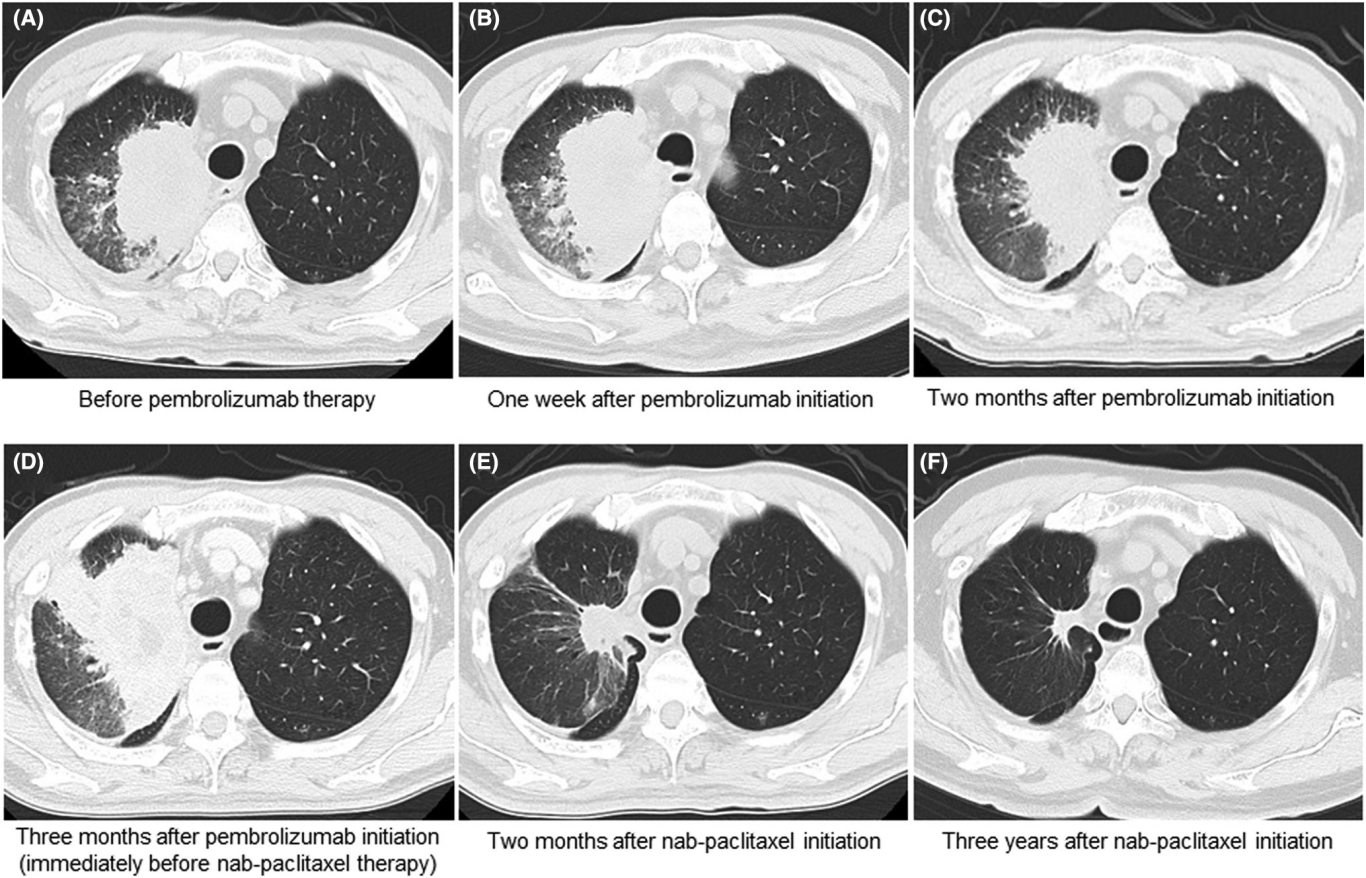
Representative CT images of a patient who showed long‐term response to nab‐paclitaxel after failure of pembrolizumab therapy. A 69‐year‐old man with an adenocarcinoma in his right upper lung lobe (A) received pembrolizumab monotherapy as a second‐line treatment. After 1 week of pembrolizumab therapy, a transient mild enlargement was observed (B), followed by a slight shrinkage 2 months later (C). However, disease progression was observed 3 months after the initiation of pembrolizumab therapy (D). He received nab‐paclitaxel immediately after pembrolizumab failure. Two months after nab‐paclitaxel monotherapy, considerable tumor shrinkage was observed (E). This response persisted until 3 years (F), with minimal adverse events, and the patients are still receiving nab‐paclitaxel therapy.
